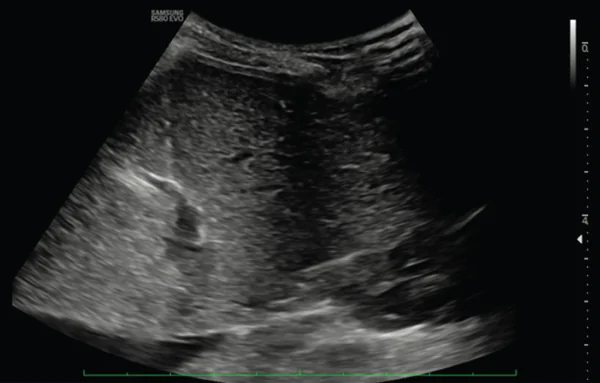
Transverse ultrasound image showing hepatic lymphatic access with a 23G needle anterior to the portal vein.
